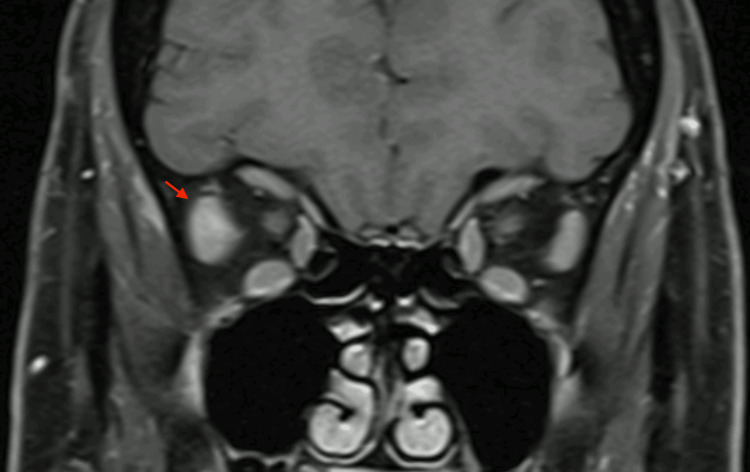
Coronal view of T1-weighted MRI test The MRI shows abnormal swelling of the right lateral rectus belly (arrow) MRI: magnetic resonance imaging
